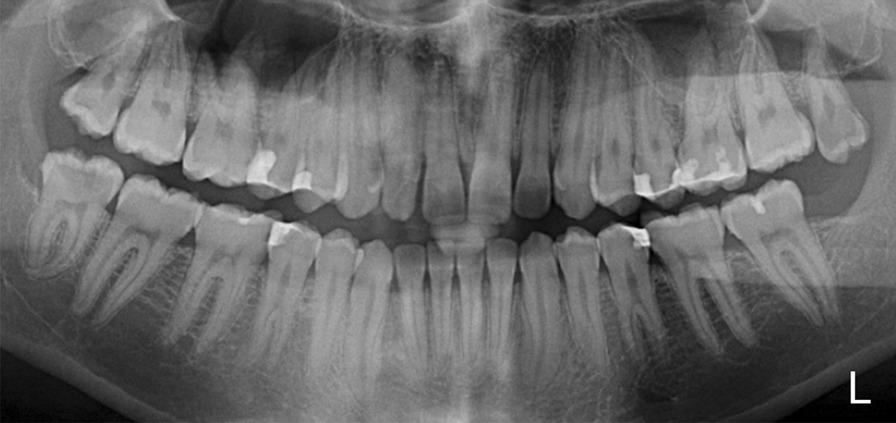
Coincidental observation in a panoramic radiograph section of a male Swiss individual depicting bilateral two-rooted mandibular second premolars. Kannan et al. [56] described a similar clinical case with contralateral two-rooted mandibular second premolars in an Indian individual. Multiple root canals can also be presumed in the second maxillary and first mandibular premolars
